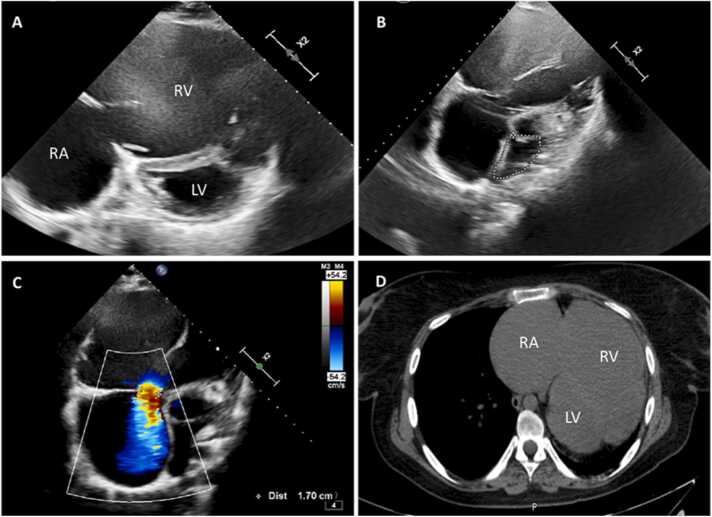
(A) Echocardiography with massive right ventricle (RV) dilation, flattening of the interventricular septum. (B) Compression of the left atrium that reduces its area to 9.9 cm^2^. (C) Severe tricuspid regurgitation due to defective leaflet coaptation, with a vena contracta measuring 15 mm. (D) Chest computed tomography revealed severe right atrium (RA) and RV dilation, associated with leftward mediastinal shift and decreased pulmonary volume.
